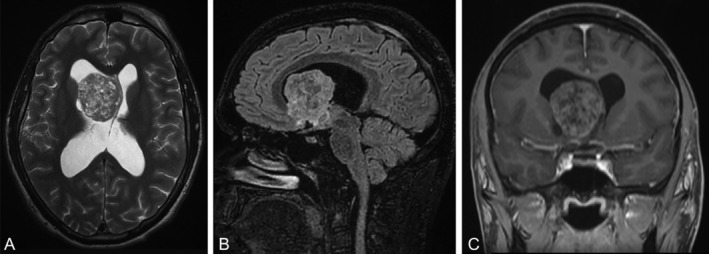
Magnetic resonance imaging findings. (A) An axial T2 image of the brain shows a heterogeneously hyperintense mass in the anterior horn of the right ventricle. (B) A sagittal FLAIR image of the brain shows a heterogeneously hyperintense mass in the anterior horn of the right ventricle. (C) A coronal T1 image of the brain shows a heterogeneously isointense mass with mild contrast enhancement in the anterior horn of the right ventricle.
